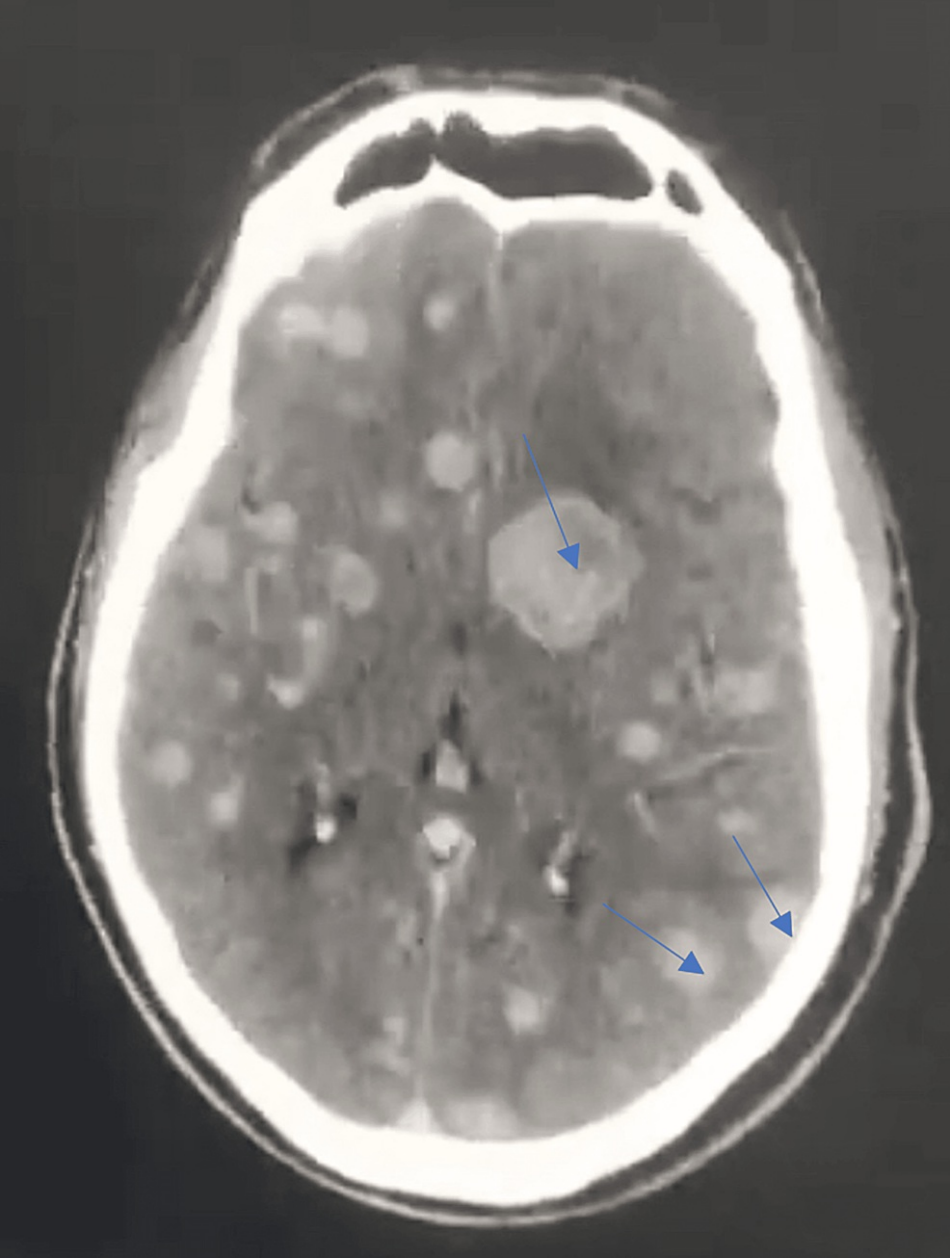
A brain CT scan revealed parenchymal nodular brain lesions without perilesional edema, resembling a balloon release (blue arrows).
